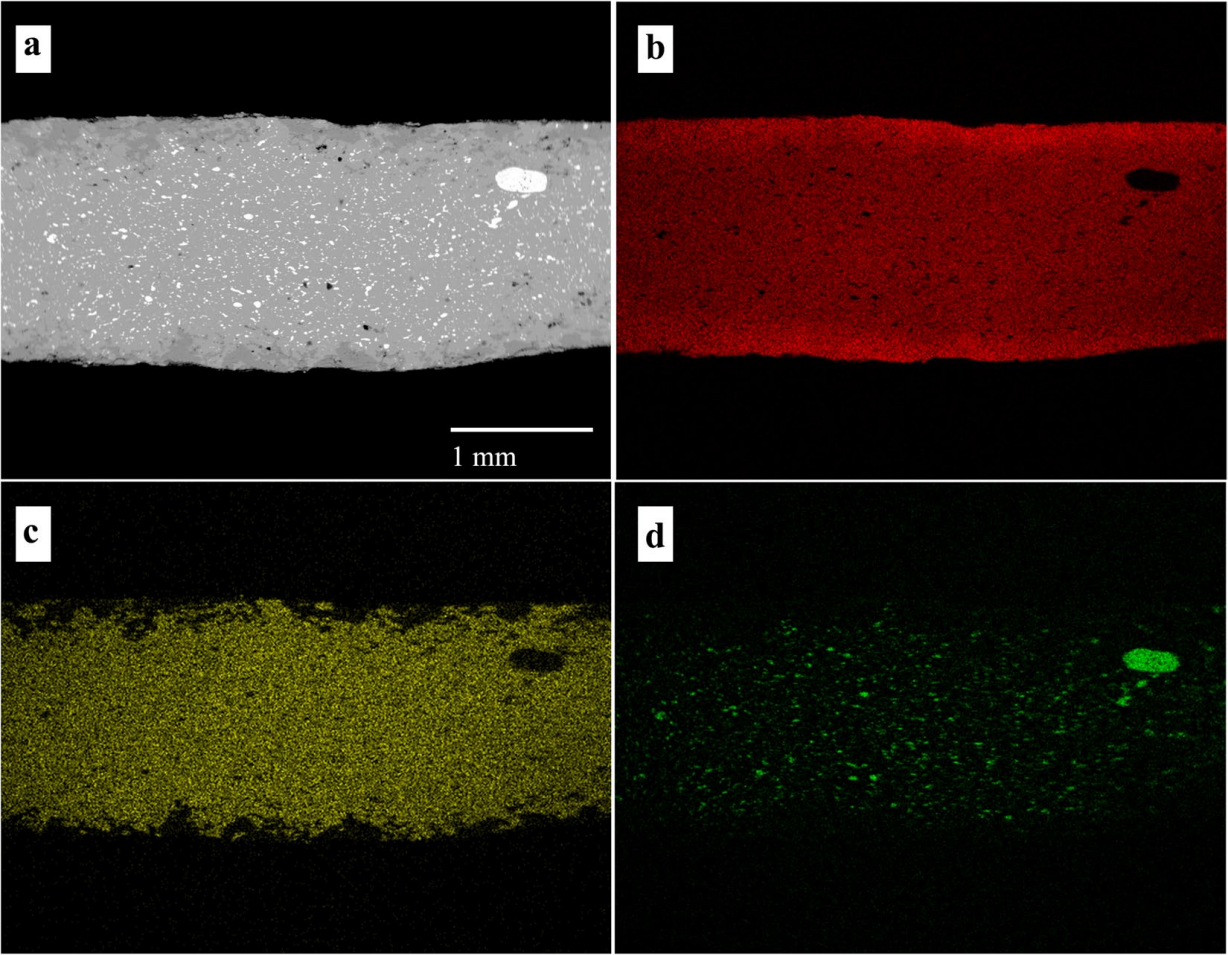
BSE image of sample B5 (a) and X-ray maps of Cu (b), Zn (c) and Pb (d).
